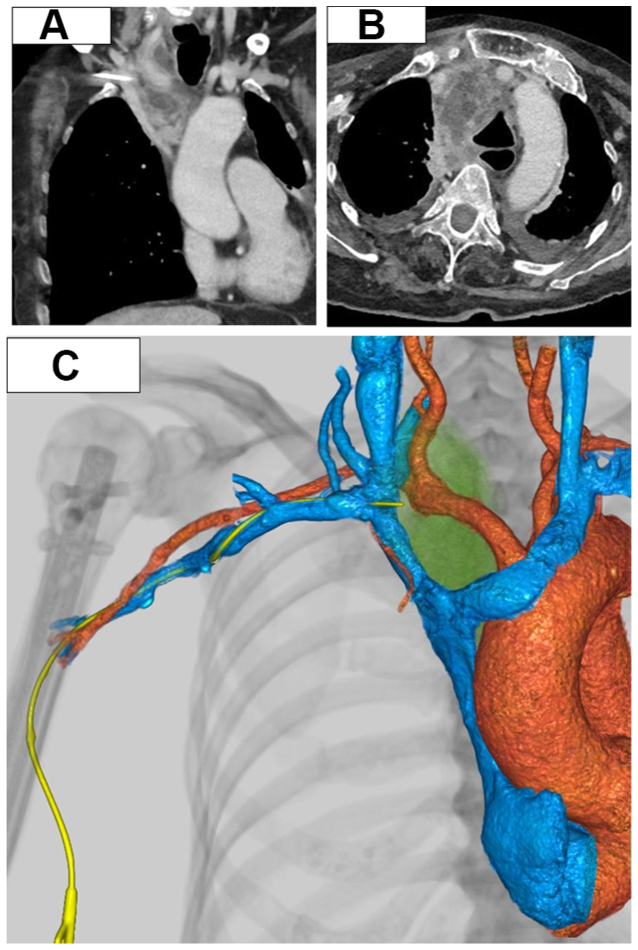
(A) Preoperative computed tomography image revealing extravascular perforation caused by PICC at the junction of the right brachiocephalic and subclavian veins. (B) Preoperative computed tomography image revealing abscess formation in the superior mediastinum. (C) Three-dimensional computed tomography image revealing extravasation of the PICC. *PICC*, peripherally inserted central catheters.
